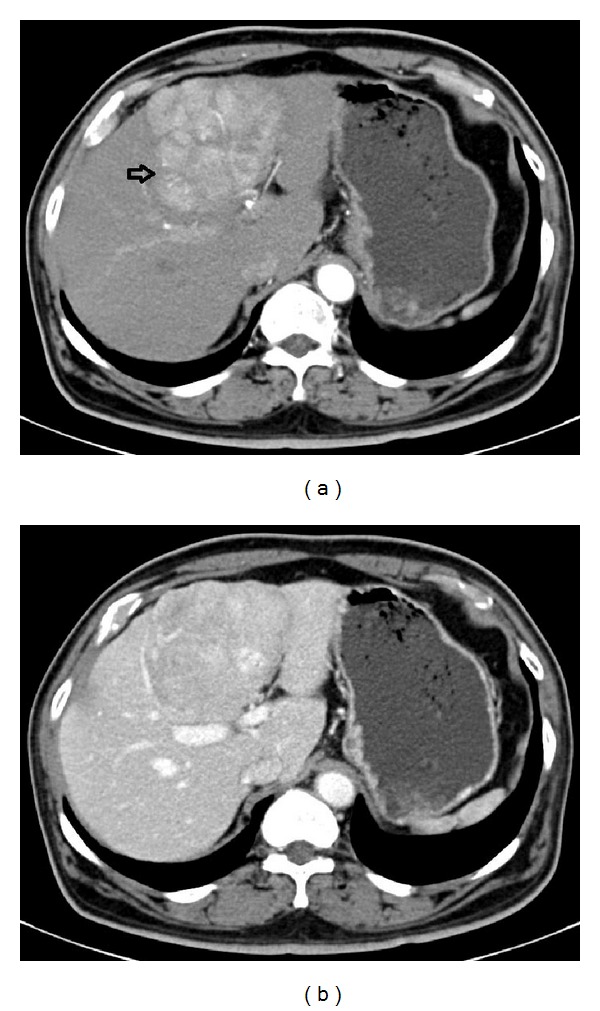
Contrast-enhanced CT scan of NASH-associated HCC. (a) This arterial-phase image shows an enhancing lesion (arrow) in the left lobe of a steatotic liver. No gross radiographic evidence of cirrhosis is present. (b) The same tumor shows hypoenhancement or “washout” in the delayed portal venous-phase image.
